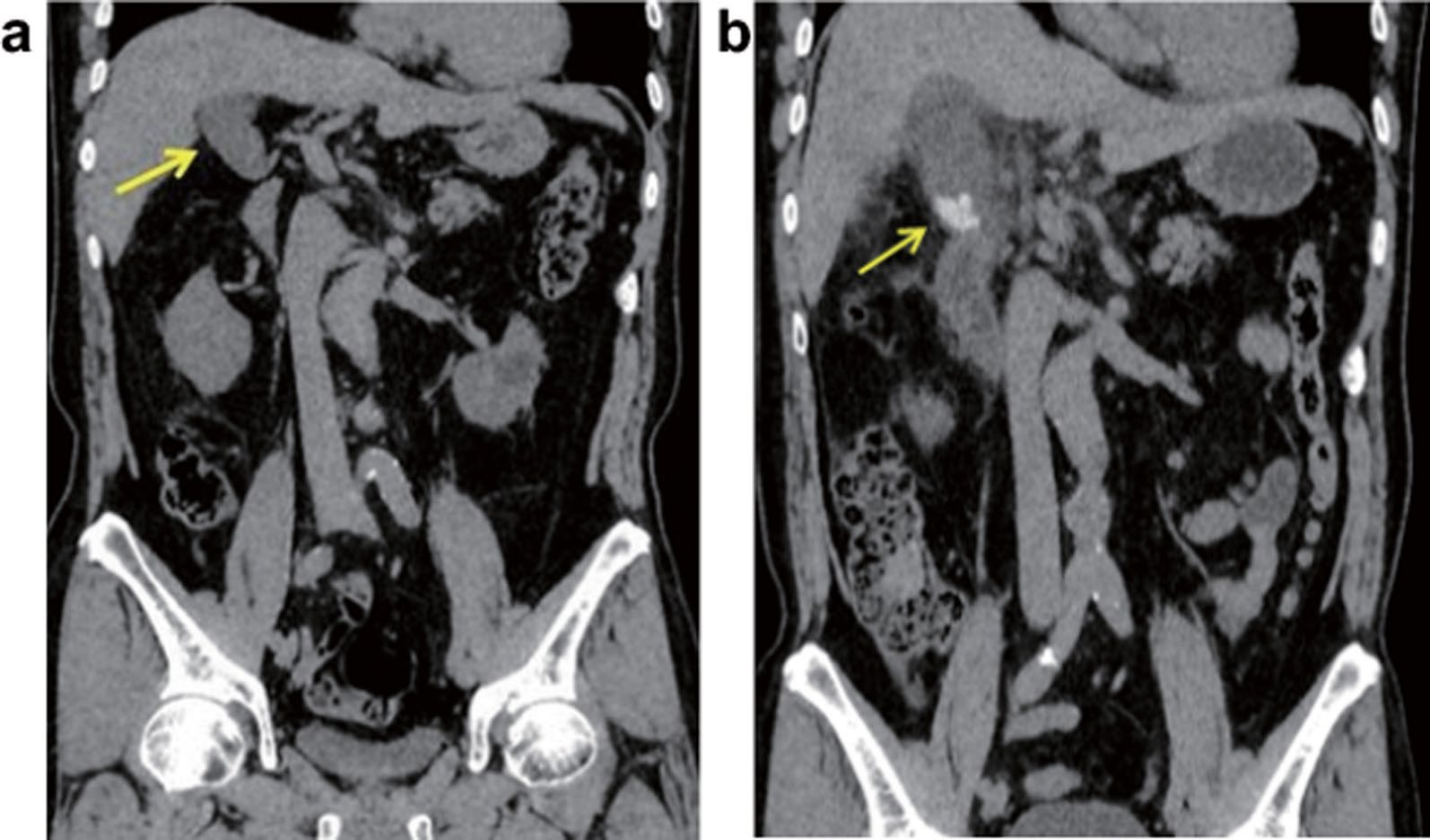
Computed tomography study. Coronal CT section before (a) and after (b) the administration of ceftriaxone. Gallbladder stone was not detected before ceftriaxone (a). However, an inflamed gallbladder (arrow) with stones can be visualized after ceftriaxone administration (b)
